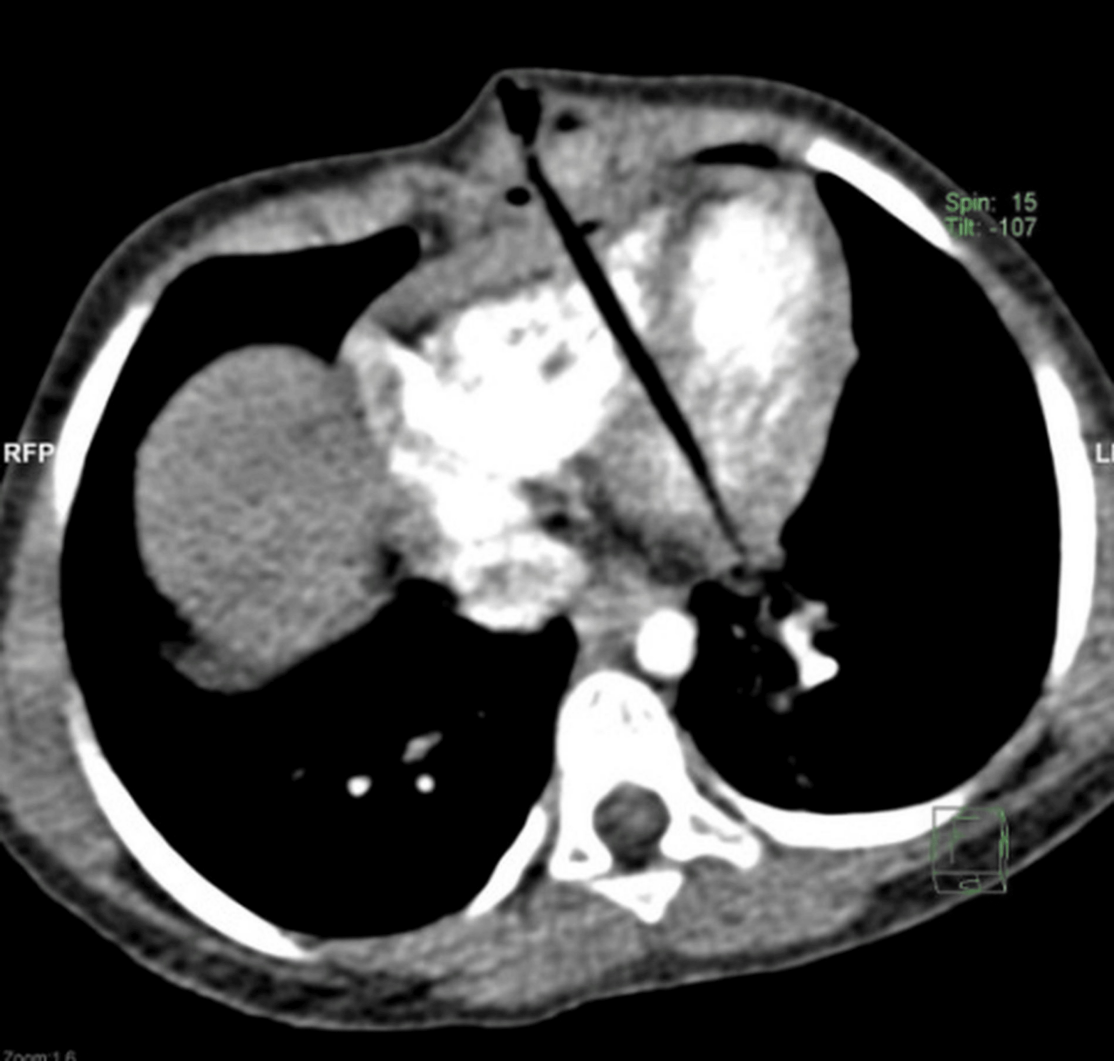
CT chest shows the thorn through the right ventricle (RV), interventricular septum (IVS) and distal tip penetrating the left ventricle (LV).
